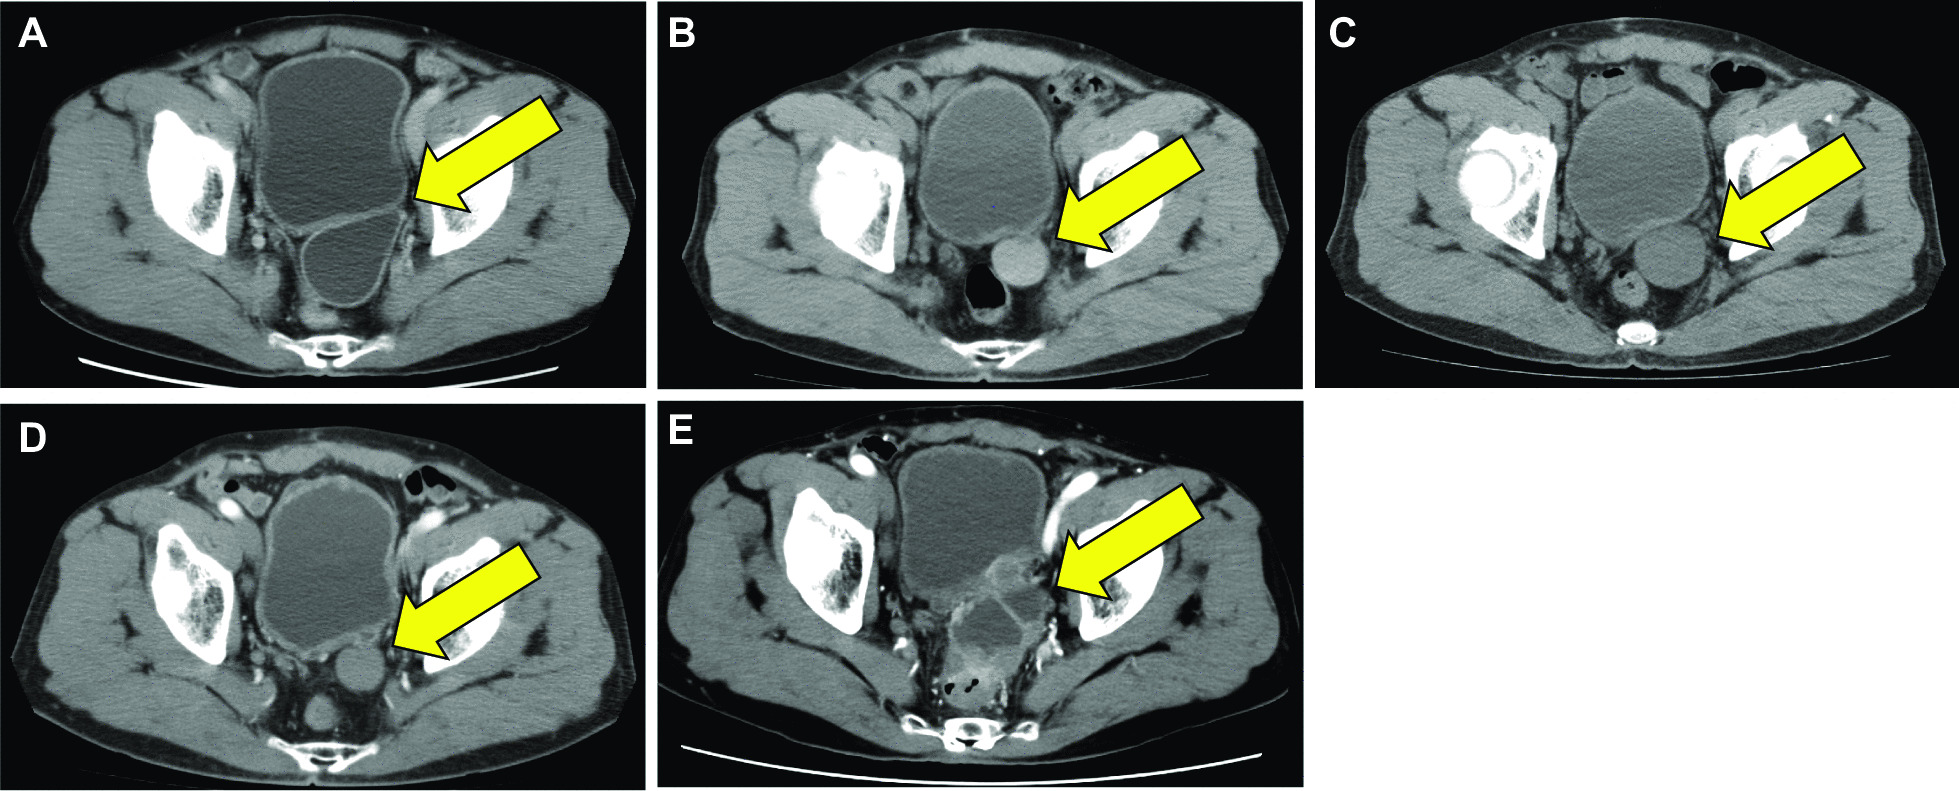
CT images before (A), 8 months (B), 3 years (C), 8 years (D), and 17 years after (E) diverticulectomy. The yellow arrows indicate the diverticular area. CT, computed tomography
